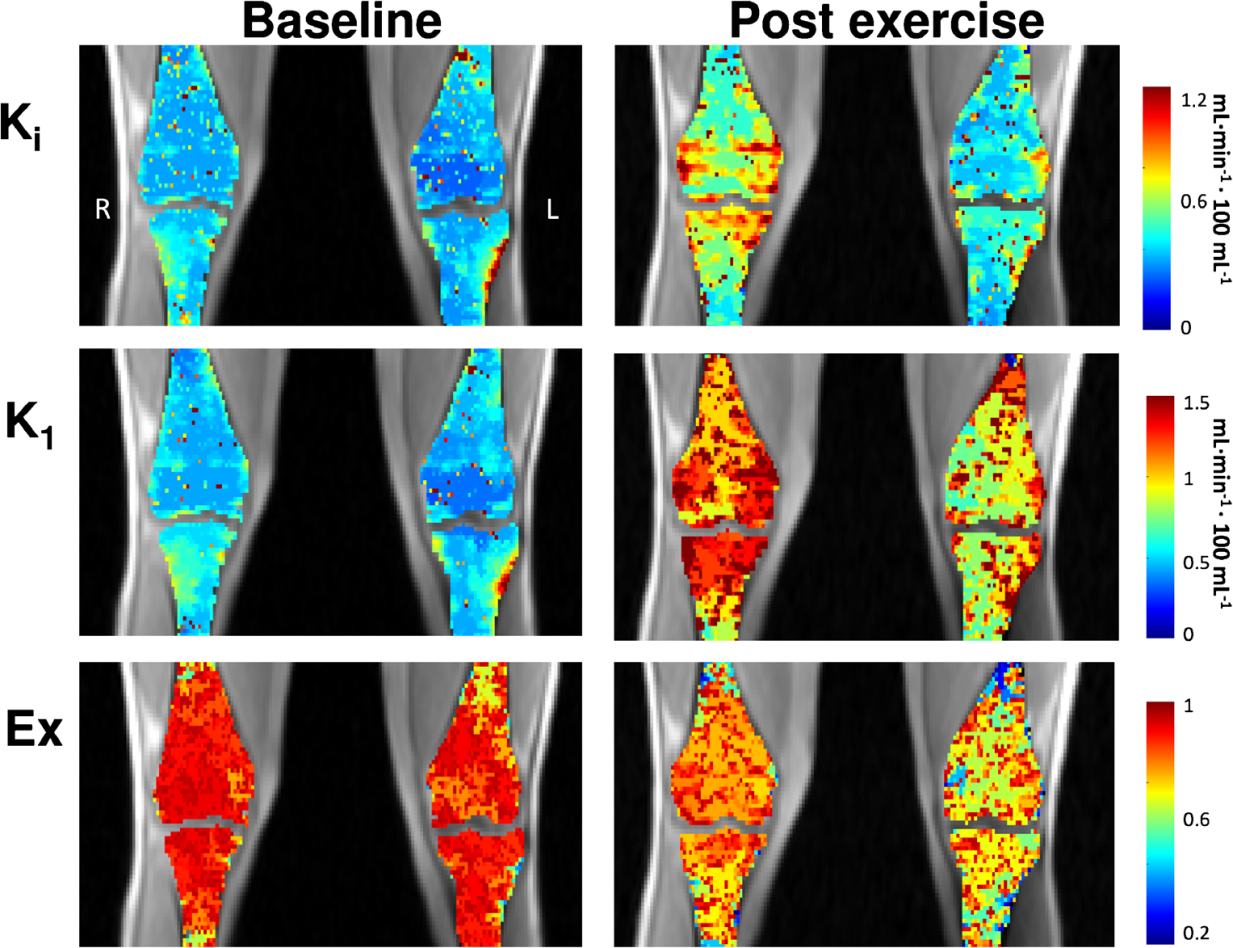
Changes in kinetic parameters after exercise in one subject. After exercise, an increase in measured K_i_, K_1_, and k_2_ values and a decrease in k_3_ values were obtained using NLR fitting. The opposing changes in k_2_ and k_3_ values both contribute to a decrease in extraction fraction (Ex) from near total extraction at baseline (k_3_/(k_2_+k_3_) ≈1) to a range of 0.35–0.8. Above are parametric maps for one subject at baseline and after exercise. Individuals had diverse patterns of activation including visible asymmetry between the step-up and drop–land leg in the post-exercise parametric maps. In subchondral bone, changes in SUV and values of all kinetic parameters, except blood volume and k_2_, varied significantly between the subregional ROIs
